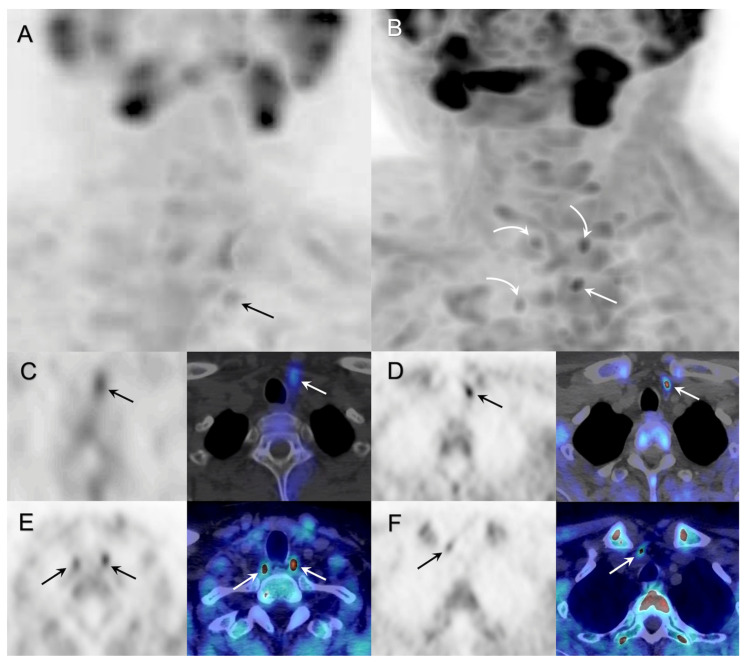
33-year-old kidney transplant recipient with tHPT. Cervical US was negative, and parathyroid scintigraphy (arrows, (A): MIP SPECT anterior image, (C): axial SPECT and SPECT/CT) suggested a hyperfunctioning left inferior parathyroid. Preoperative 18F-FCholine PET/CT showed increased focal uptake in all four parathyroids (arrows, (B): MIP PET anterior image, (D–F): axial PET and PET/CT). The patient underwent bilateral neck exploration as well as left superior and left inferior parathyroidectomy. Pathology showed parathyroid hyperplasia in both glands. The reduction of preoperative PTH serum concentration was 60%, and the patient presented with recurrent HPT about 12 months after surgery.
